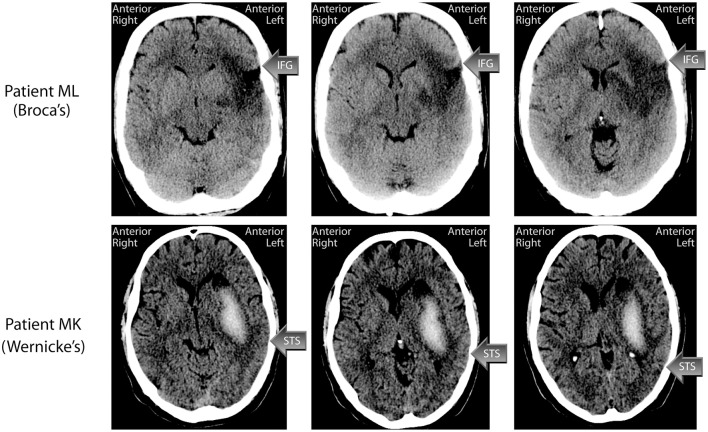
CT-scans of the lesions of patients ML (Broca's aphasic) and MK (Wernicke's aphasic). Arrows indicate the approximate location of the inferior frontal gyrus (IFG) in Patient ML and the superior temporal sulcus (STS) in Patient MK. See main text for a description of the patients' lesions.
